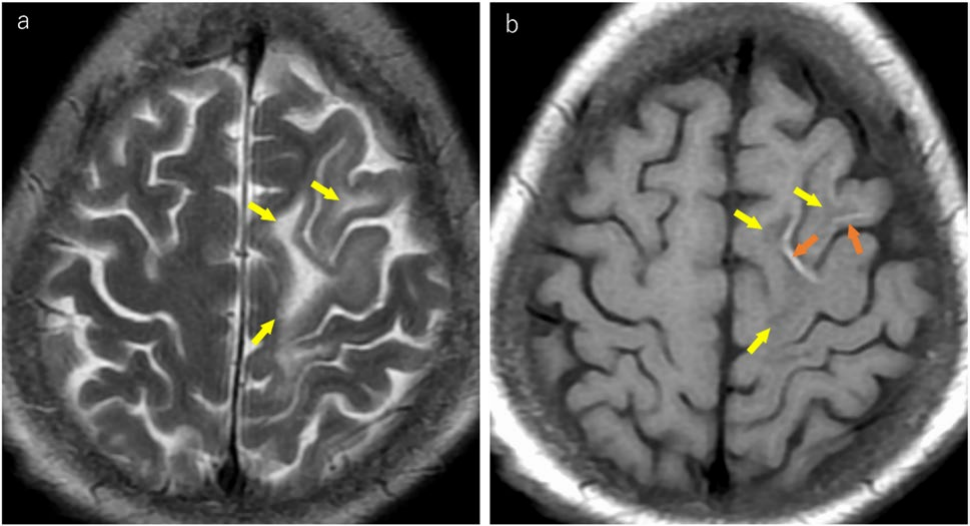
Woman in her 70 s with severe instability and anemia was diagnosed with diffuse large B-cell lymphoma (DLBCL). Subsequently, the patient underwent chemotherapy. After the treatment, the patient presented with right-sided hemiparesis. The patient also exhibited progressive articulatory disorders and decreased swallowing function. JCV was detected in the cerebrospinal fluid, confirming a diagnosis of PML. a T2-weighted image revealing hyperintense lesions in the subcortical white matter of the left frontal lobe (yellow arrows). b On T1-weighted imaging, lesions appear hypointense (yellow arrows). The adjacent cortex shows hyperintensity (orange arrows)
